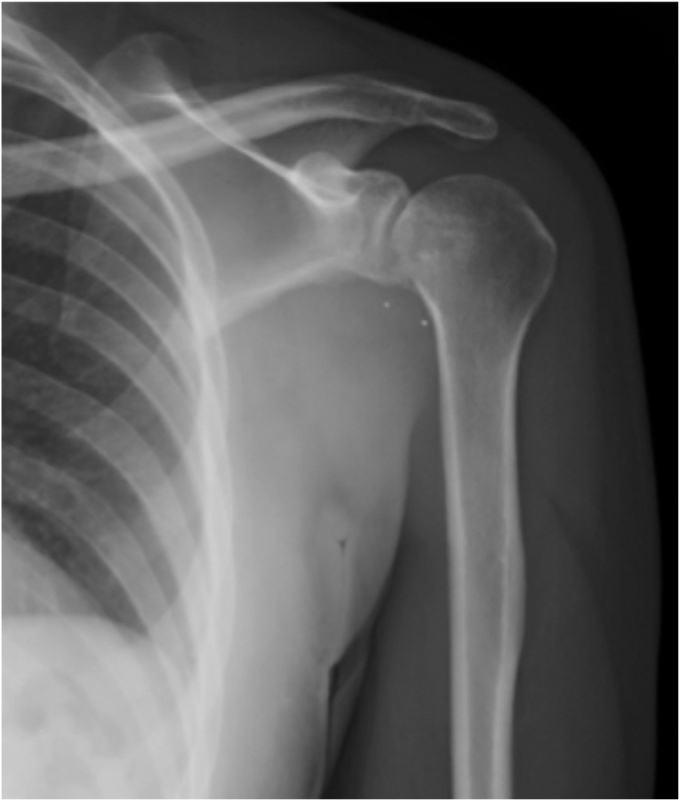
Stable appearing OCA on radiographic imaging at 2 weeks postoperative visit. *OCA*, osteochondral allograft transplantation.
